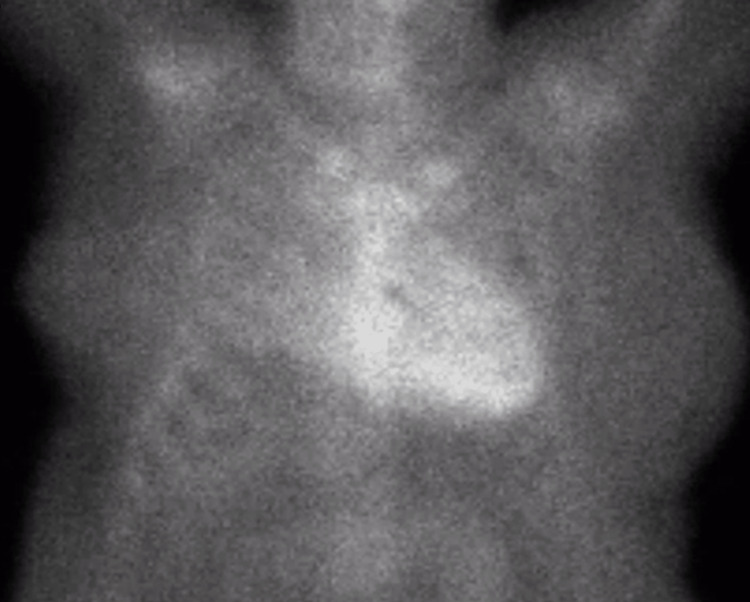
99m-Technetium-pyrophosphate scintigraphy Showing a heart-to-contralateral lung ratio of 1.76 at three hours
